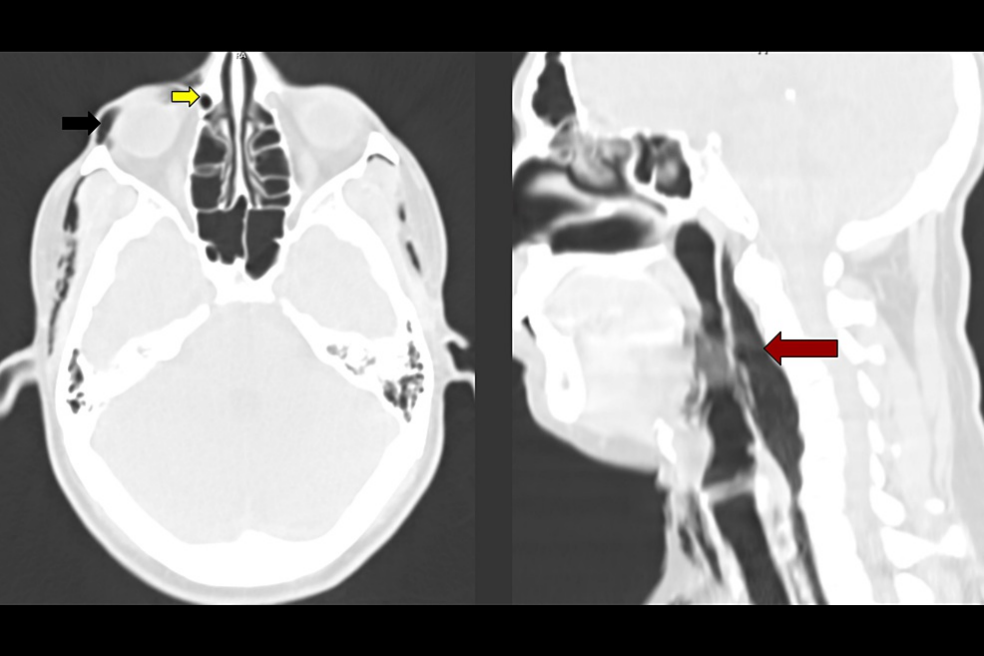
(Left) CT scan of head day 3: axial slice showing right palpebral subcutaneous emphysema (black arrow) and air in the right nasolacrimal duct (yellow arrow). (Right) CT scan of head and neck day 3: sagittal slice showing air dissecting posterior to the pharynx (red arrow) and resultant displacement of posterior structures anteriorly.
